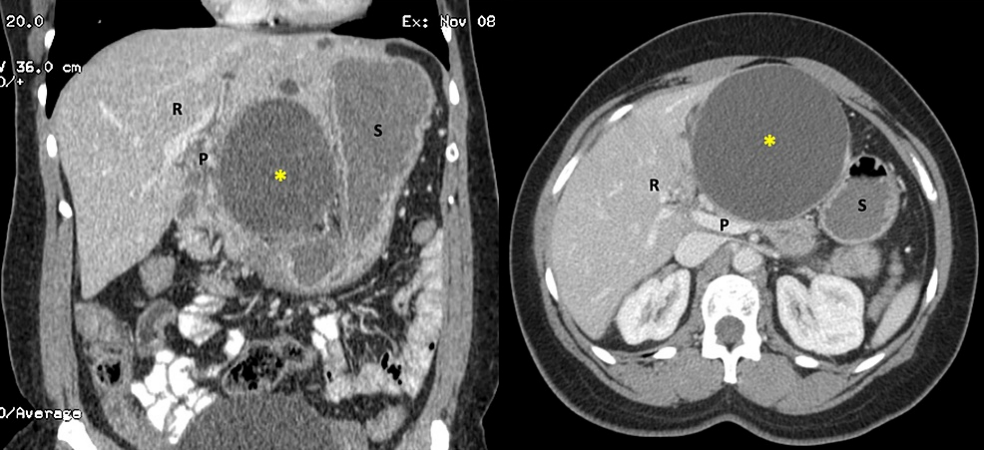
CT scan images revealing a large, ruptured left hepatic cyst. The large 12x15 cm ruptured hepatic cyst (asterix) is visible. The stomach (S) is draped over the cyst to contain the rupture along with the right liver (R).
